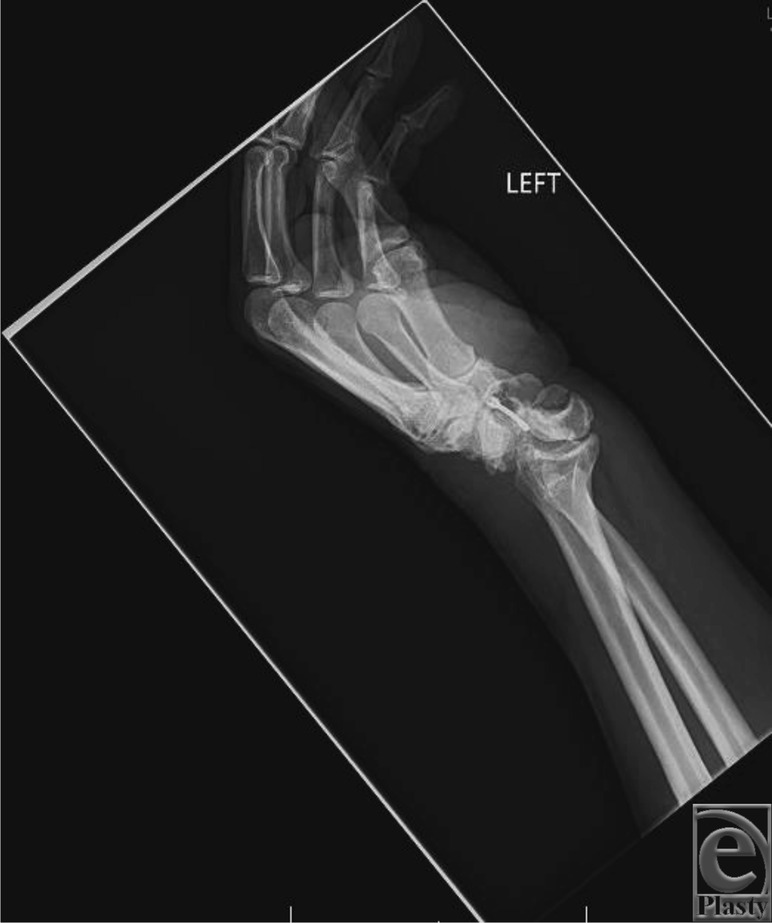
Preoperative lateral radiograph of transscaphoid transcapitate perilunate fracture-dislocation in the setting of scaphoid nonunion and scaphoid nonunion advanced collapse demonstrating posterior displacement of the capitate relative to the lunate.
